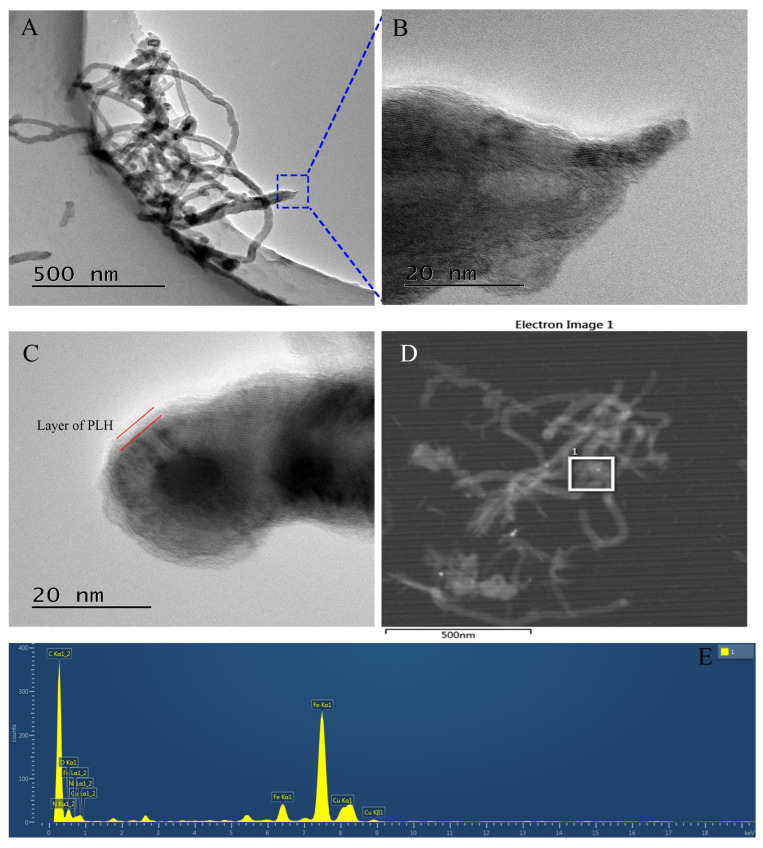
TEM images of MWCNTs (A), CMWCNTs (B), PLH-CMWCNTs (C), HADDF image (D) at different magnifications and EDX spectrum (E) of Fe(III)-PLH-CMWCNTs. Materials were dispersed by ultrasound, and the samples were made of 3 mm (200 mesh) copper mesh with a microgrid carbon film.
